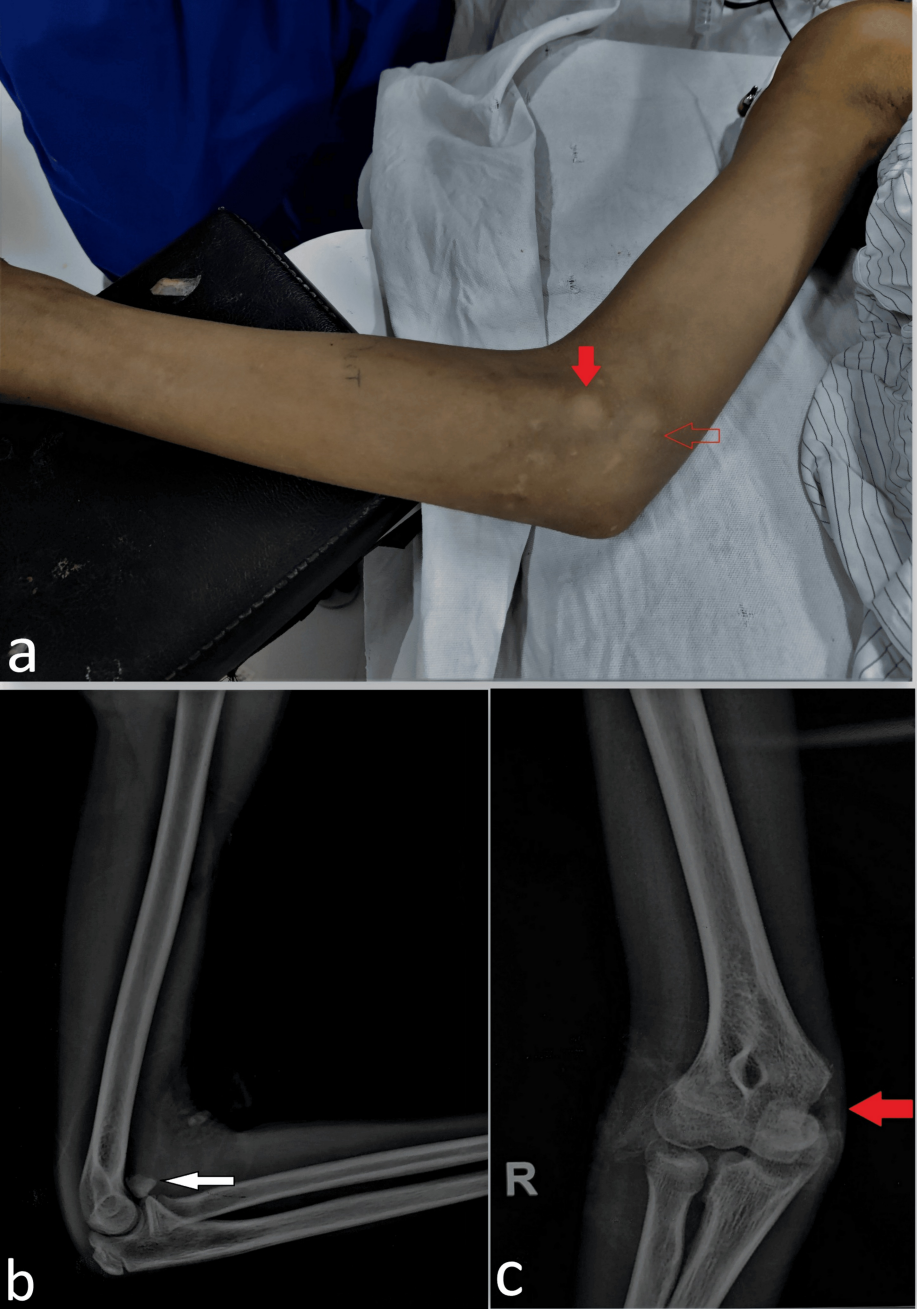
The clinical image (a) shows the presence of a double medial bump by the displaced medial epicondyle fragment placed anteriorly (solid red arrow) and the native humeral medial condyle bed situated posteriorly (hollow red arrow). The elbow radiograph at presentation shows a bony fragment anterior to the elbow joint (denoted by a white arrow) in the lateral view (b), and a defect at the normal location of the medial epicondyle region (c), suggesting an absence of the medial epicondyle from that area (denoted by a thicker red arrow).
